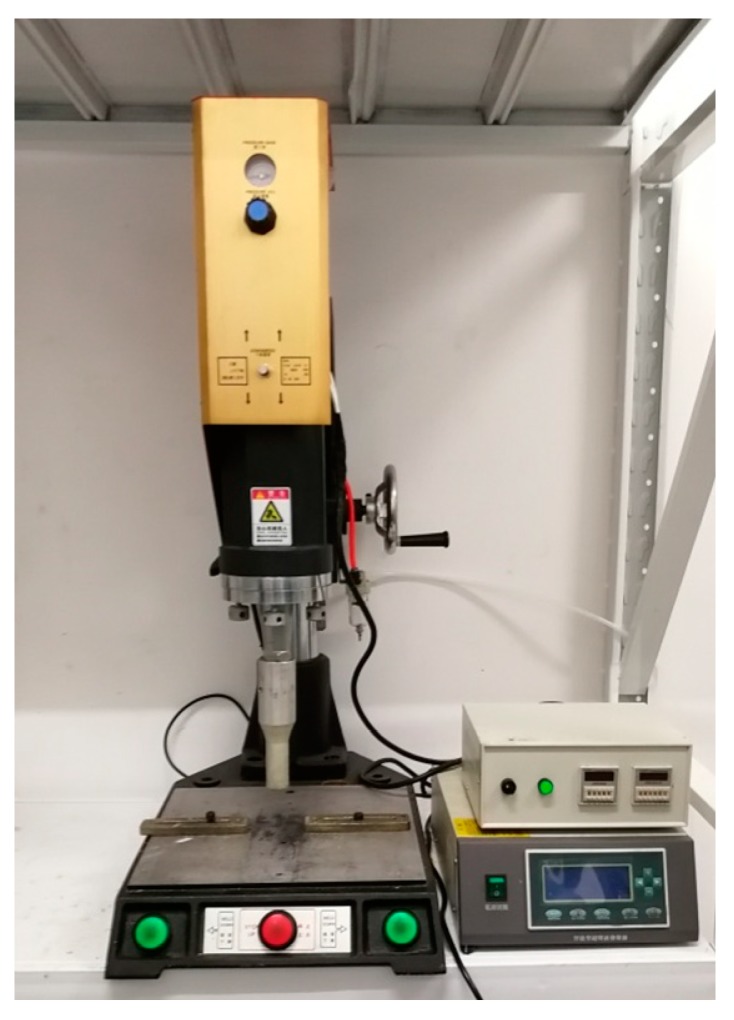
Ultrasonic experiment platform.
